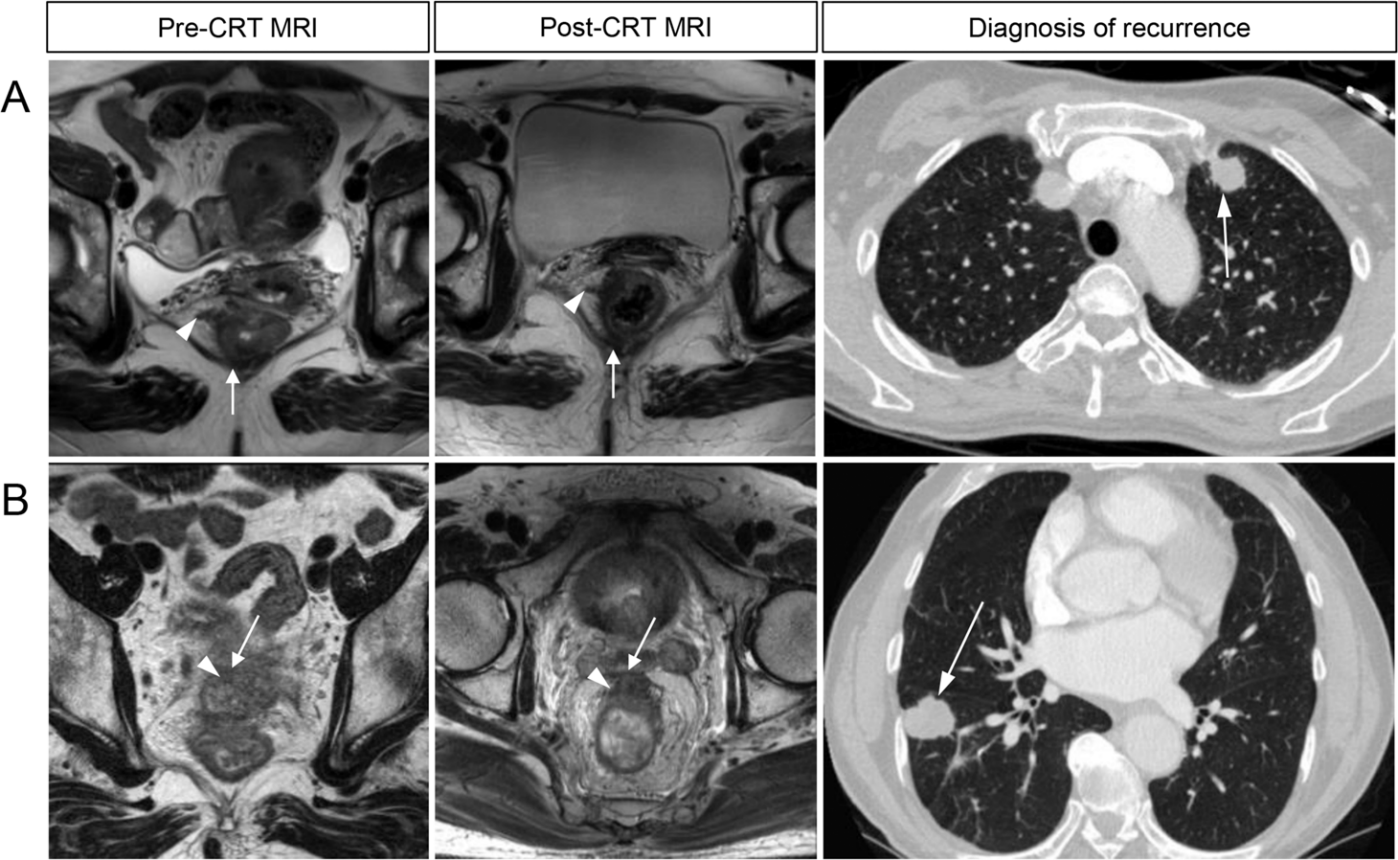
Representative images of a pre-CRT MRI (left column), post-CRT MRI (middle column), and image of a recurred tumor obtained from a pCR patient who later developed tumor recurrence. (A) 50-year-old female with lung metastasis (relapse-free period, 22.9 months), (B) 70-year-old male with lung metastasis (relapse-free period, 37.4 months). Positive MRI mesorectal fascia (short arrow), positive MRI extramural vessel invasion (arrowhead), and a recurred tumor lesion (long arrow) are depicted.
